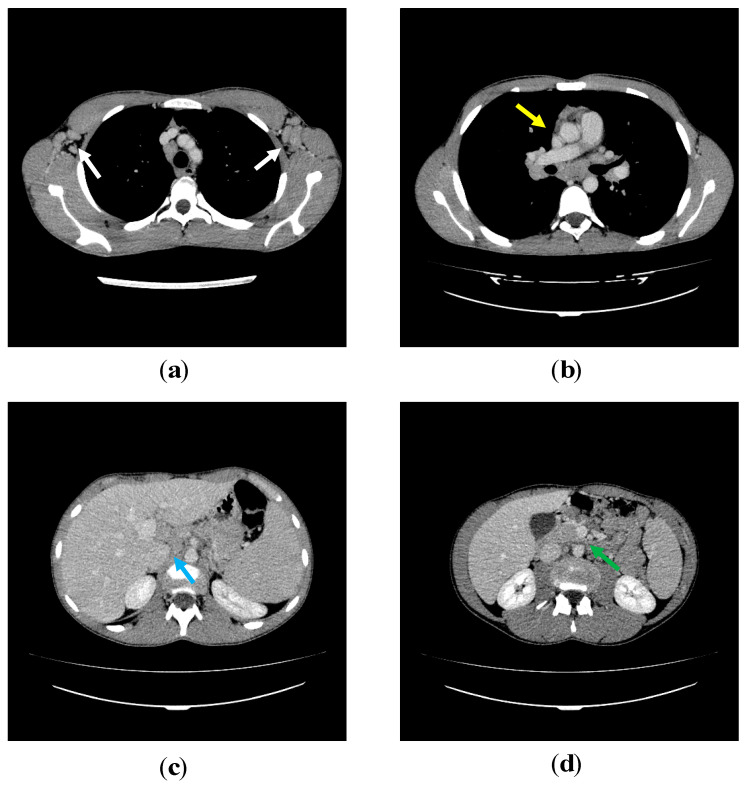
P2′s thoraco-abdominal computed tomography (CT) scans. On post contrast axial CT scans at the level of the axillary regions (a) numerous lymph nodes (white arrows) with homogeneous density are evident. On the scan just below the tracheal carina (b); solid mediastinal adenopathic conglomerate and bilateral hilar lymph nodes (yellow arrow) are clearly visible. In the abdomen, multiple adenopathies are evident in the hepatic hilum (c) (blue arrow) and in the mesentery (d) (green arrow).
